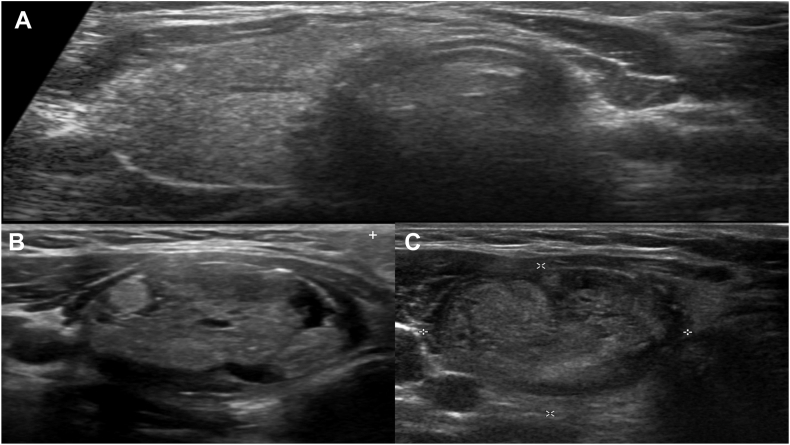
Postoperative thyroid ultrasound. *A*, The transverse view of the thyroid ultrasound shows a clear *left* thyroid bed following a *left* thyroid lobectomy. *B*, Baseline *right* thyroid nodule (32 mm)—isoechoic, mixed cystic and solid, wider than tall, with a smooth margin and punctate echogenic foci (TIRADS-4). *C*, Two-year follow-up of the *right* thyroid nodule (32 mm)—hypoechoic, solid, wider than tall, with a smooth margin and punctate echogenic foci and echogenic foci (TIRADS-5). *TIRADS* = Thyroid Imaging Reporting and Data System.
